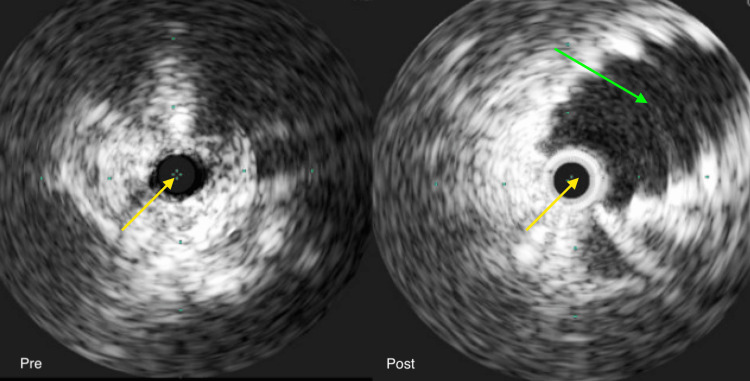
Intravenous ultrasound showed a collapsed left iliac vein pre-intervention and an expanded left iliac vein (green arrow) post-intervention. The yellow arrows are pointing out the ultrasound probe. Green dots are measurement markers.
